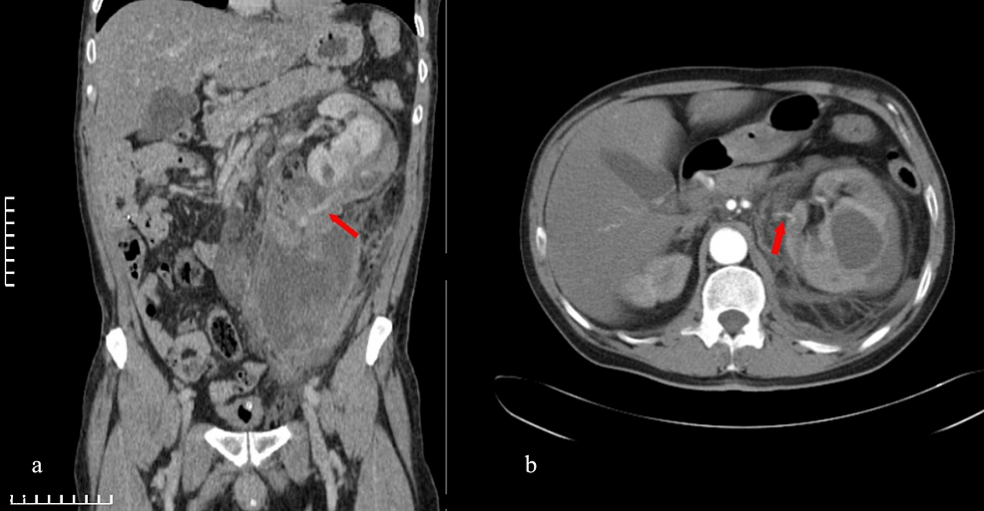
Renal rupture with active retroperitoneal bleeding and hematoma (a, b). Grade IV renal rupture on the left side according to the latest American Association for the Surgery of Trauma (AAST) renal injury grading scale [6]. The red arrows show active bleeding. (a) CT, arterial phase, coronal view, abdomen. (b) CT, arterial phase, horizontal view.
